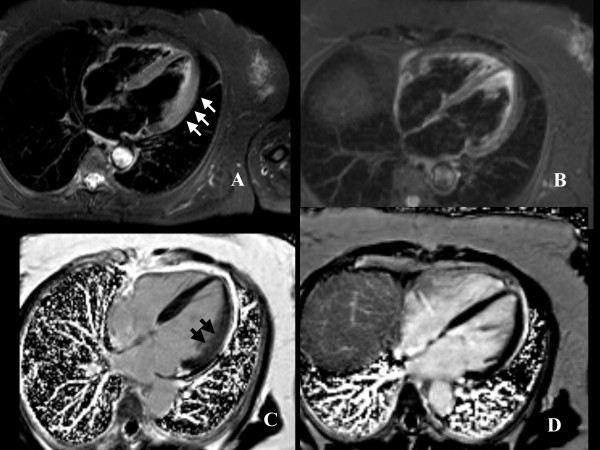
STIR and LGE images. A, four chamber view, T2-weighted image showing thickening of lateral wall with edema (white arrows); B, post-surgical follow-up T2-weighted images showing normalization of wall thickness and regression of edema; C, post-contrast images showing diffuse myocardial enhancement (black arrows); D, post-surgical follow-up post-contrast images showing regression of myocardial enhancement.
